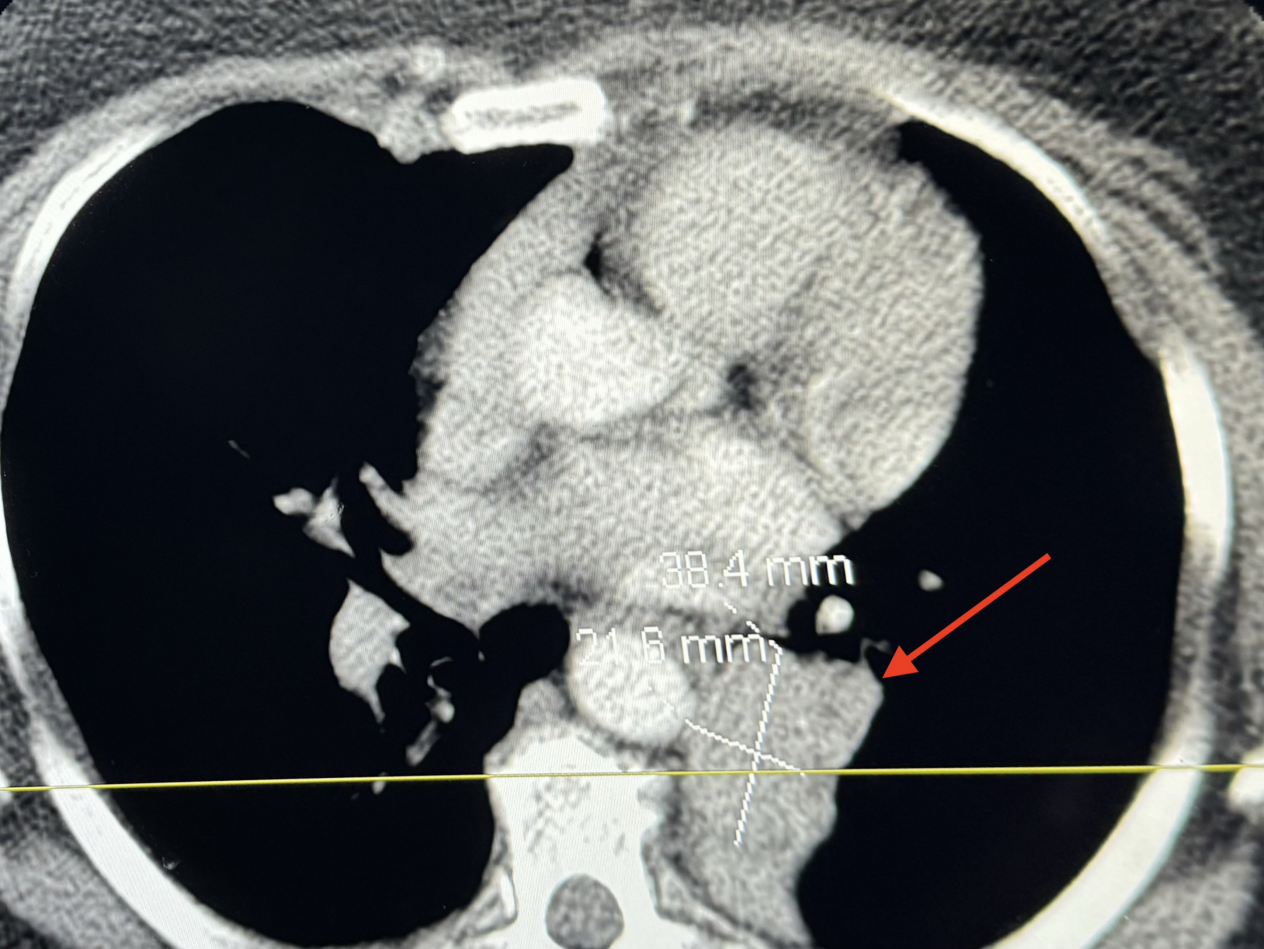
Axial CT scan showing a 38.4 mm × 21.6 mm tumor in the left lower lobe (arrow)
